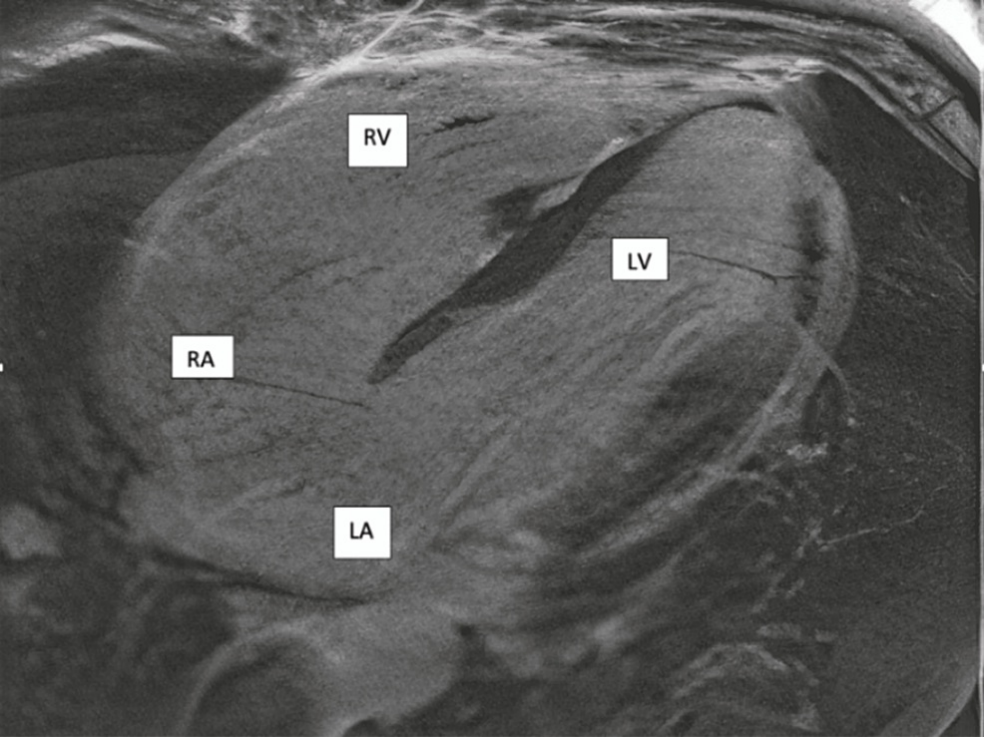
Cardiac MRI featuring marked dilatation of the right atrium (RA) and right ventricle (RV); showing left ventricle (LV) and left atrium (LA) for comparison.
